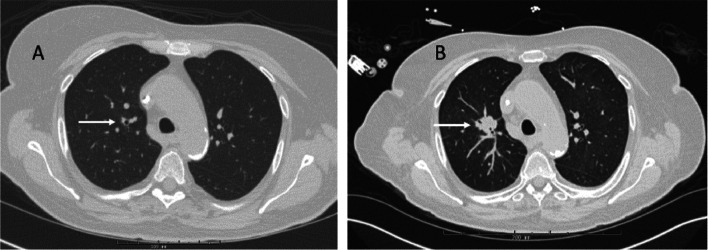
CT chest. Non-contrast computerized tomography (CT) showing right upper lobe nodular opacity 20 days before transplant, measuring 0.4 cm (A), and on day + 6 after transplant, measuring 1.6 × 1.8 cm (B). The pre-transplant opacities were of indeterminate significance, and attributed to possible resolving infection
